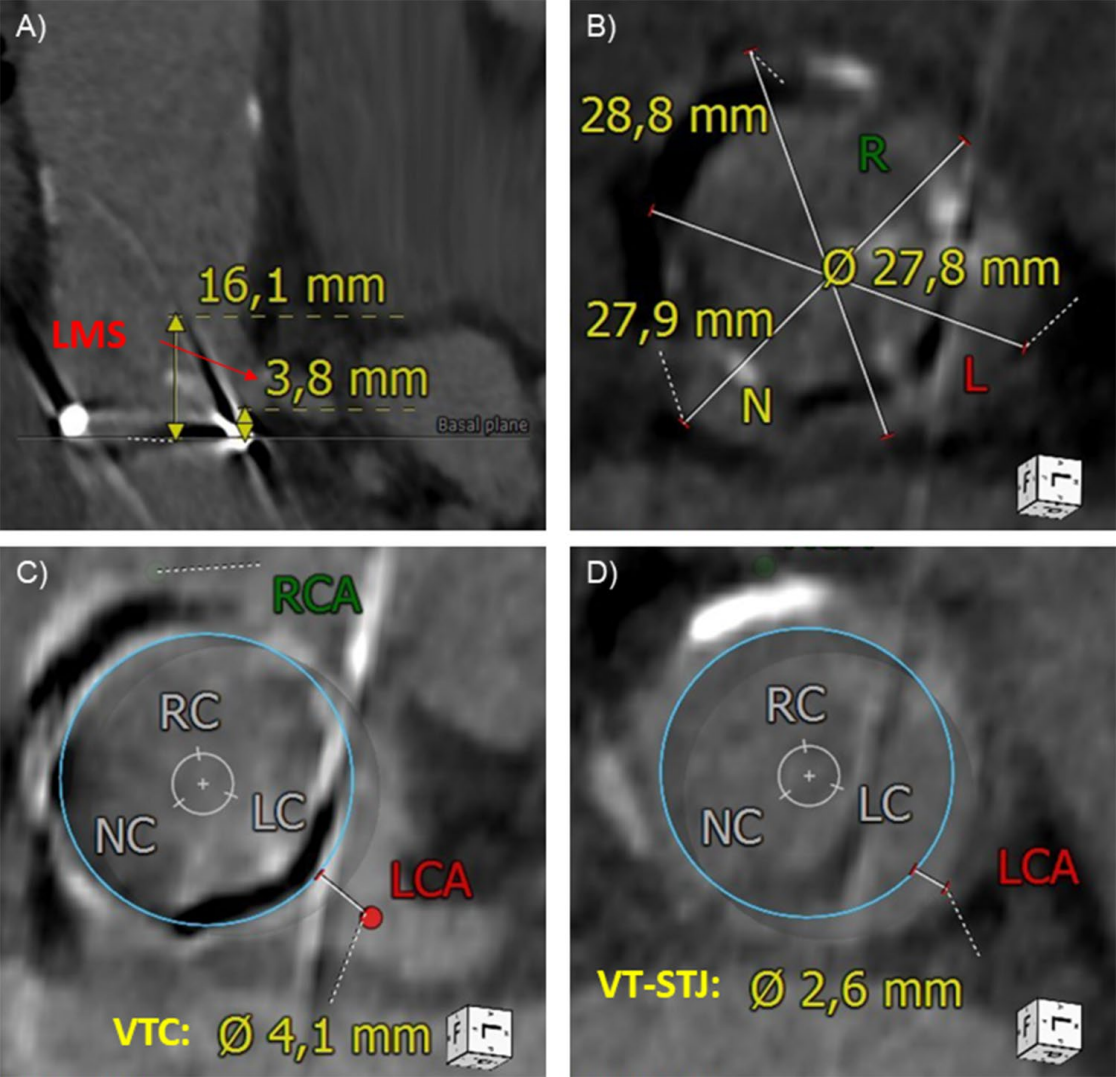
Computed tomography. Low LMS take-off, low STJ height (a) and narrow SOV (b). Relationship between a virtual THV and the LMS (c) and STJ (d). *LMS* left main stem, *LCA* left coronary artery, *RCA* right coronary artery, *STJ* sinotubular junction, *THV* transcatheter heart valve, *VTC* distance between the virtual THV and the coronary ostium, *VT-STJ* distance between the virtual THV and the sinotubular junction
